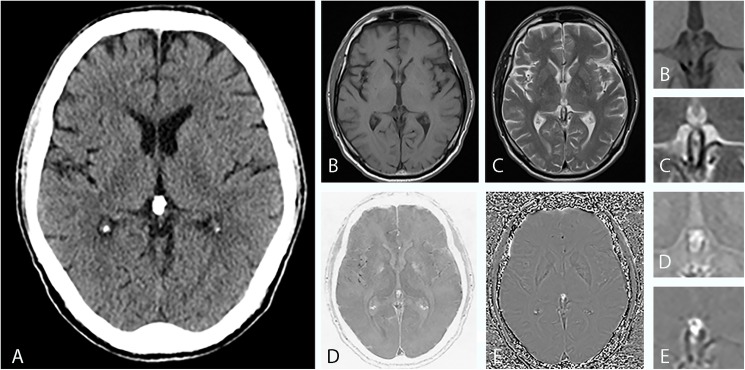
Imaging findings of a 63-year-old man with a calcified pineal gland. (A), CT shows a sharply defined oval-shaped pineal calcification with a diameter of 7 mm. In axial T1-weighted MRI (B) and in axial T2-weighted MRI (C) it is hardly possible to demarcate the calcified area against the surrounding tissue. In conventional MRI, it is not possible to reliably identify the hypointense foci as calcifications. The inverted SWMR magnitude image (D) and the phase image (E) show well-defined focal hyperintensities in the pineal region area. While the image information solely derived from the magnitude image is not superior to conventional MRI sequences, the combination of SWMR magnitude and phase image allows for a clear and reliable identification of diamagnetic calcifications. Magnified images are provided for (B), (C), (D) and (E). As CT and MRI of the brain have diverged reference lines with the bicommissural line used as a convenience standard for MRI and the orbitomeatal line used for CT, the slice angles vary slightly.
